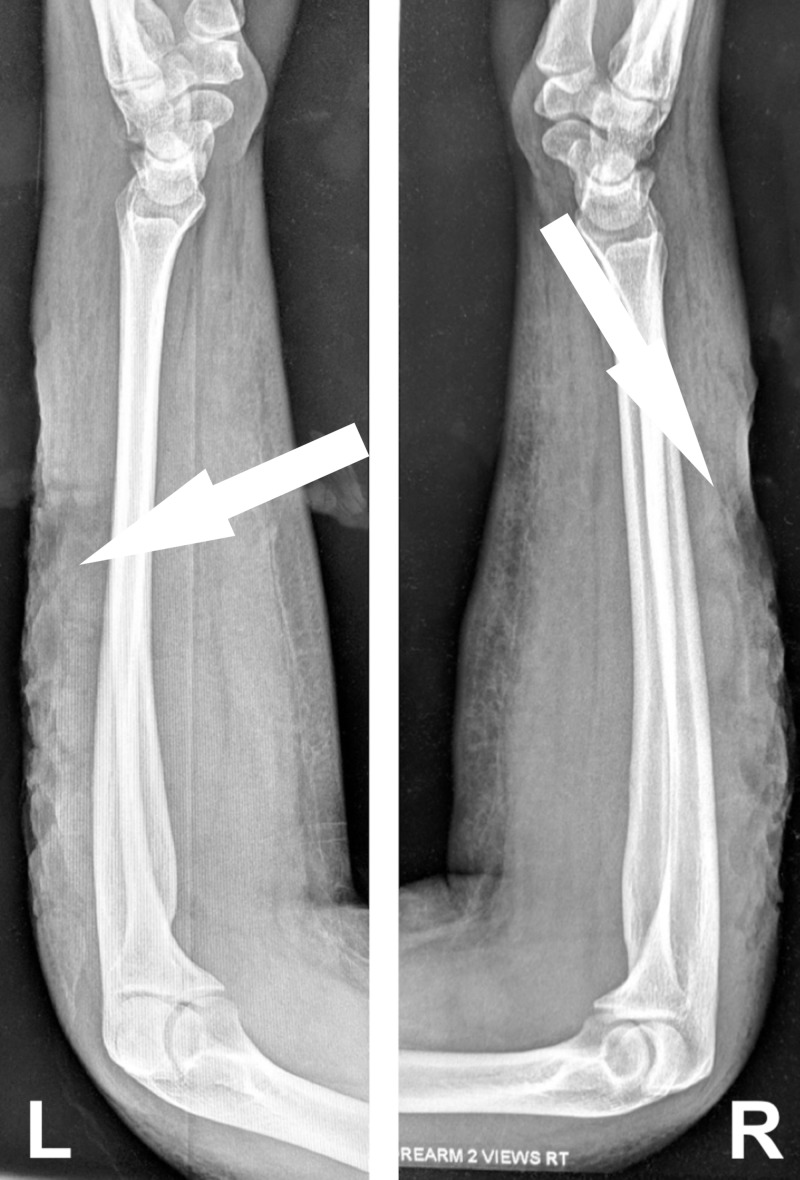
Radiographs of the forearms demonstrating gas within the soft tissue.
